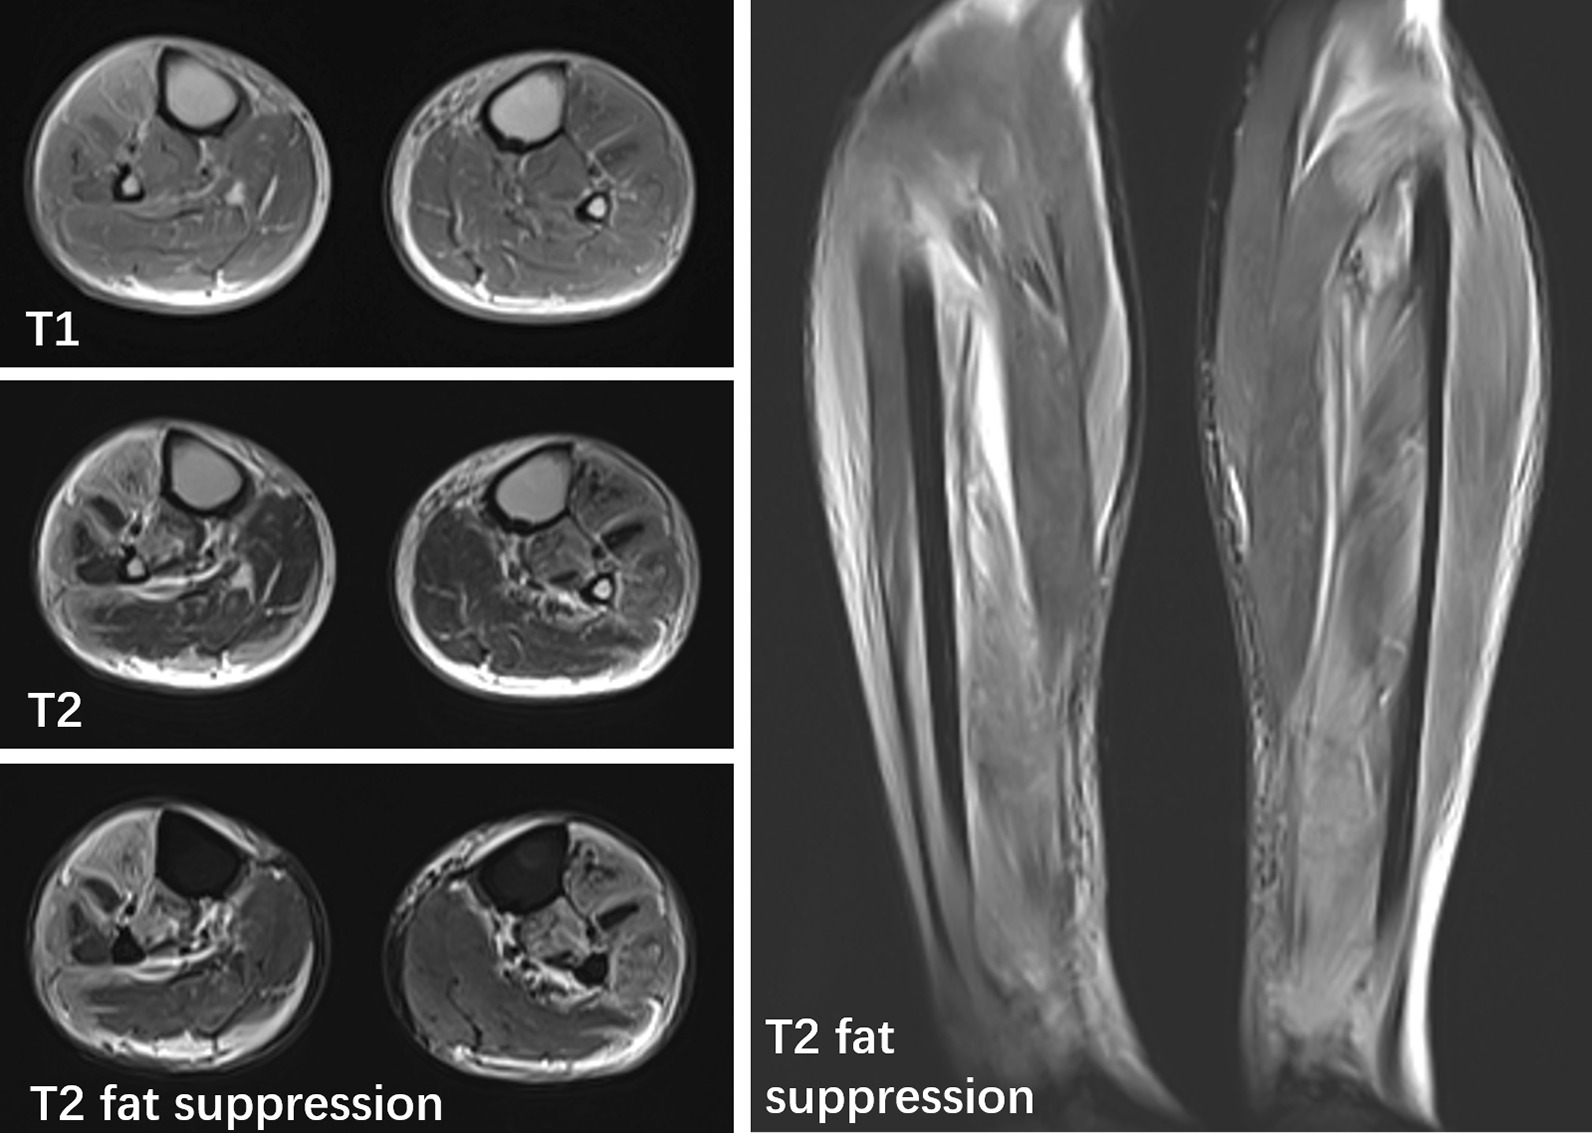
MRI showing extensive swelling in the soft tissue of the lower legs. *MRI* magnetic resonance imaging
